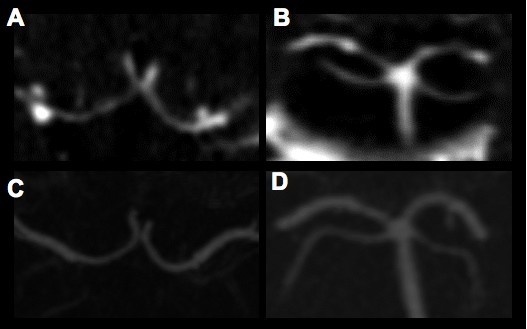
RCVS on computed tomography angiography. CTA obtained during hospitalization showed multifocal segmental vasoconstrictions most prominent in the bilateral anterior (A) and posterior (B) cerebral arteries. Follow-up CTA six weeks after discharge revealed marked resolution of cerebral artery vasoconstriction, shown here for anterior (C) and posterior (D) cerebral arteries.
